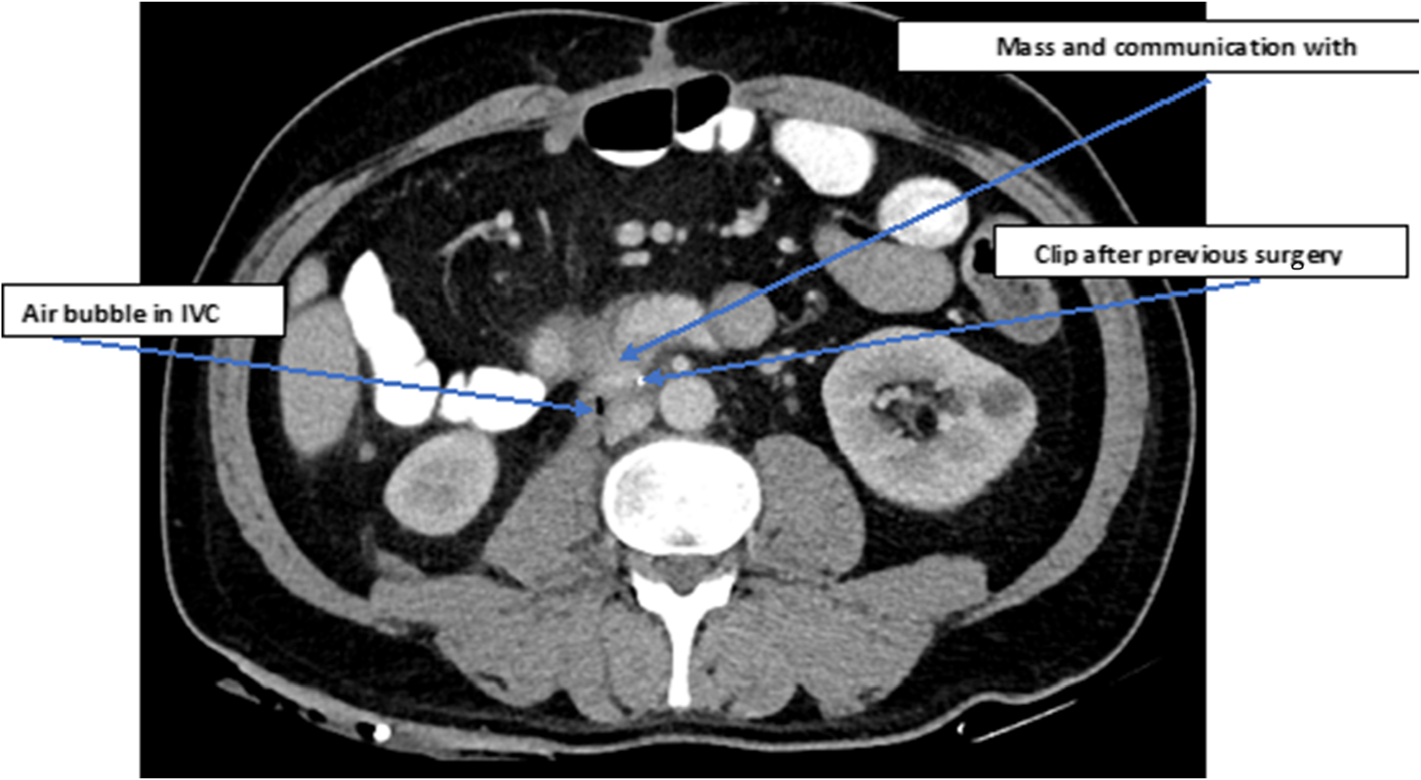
Abdominal CT. The mass shown with *arrows* indicating its connection to the IVC and clips from previous surgery
